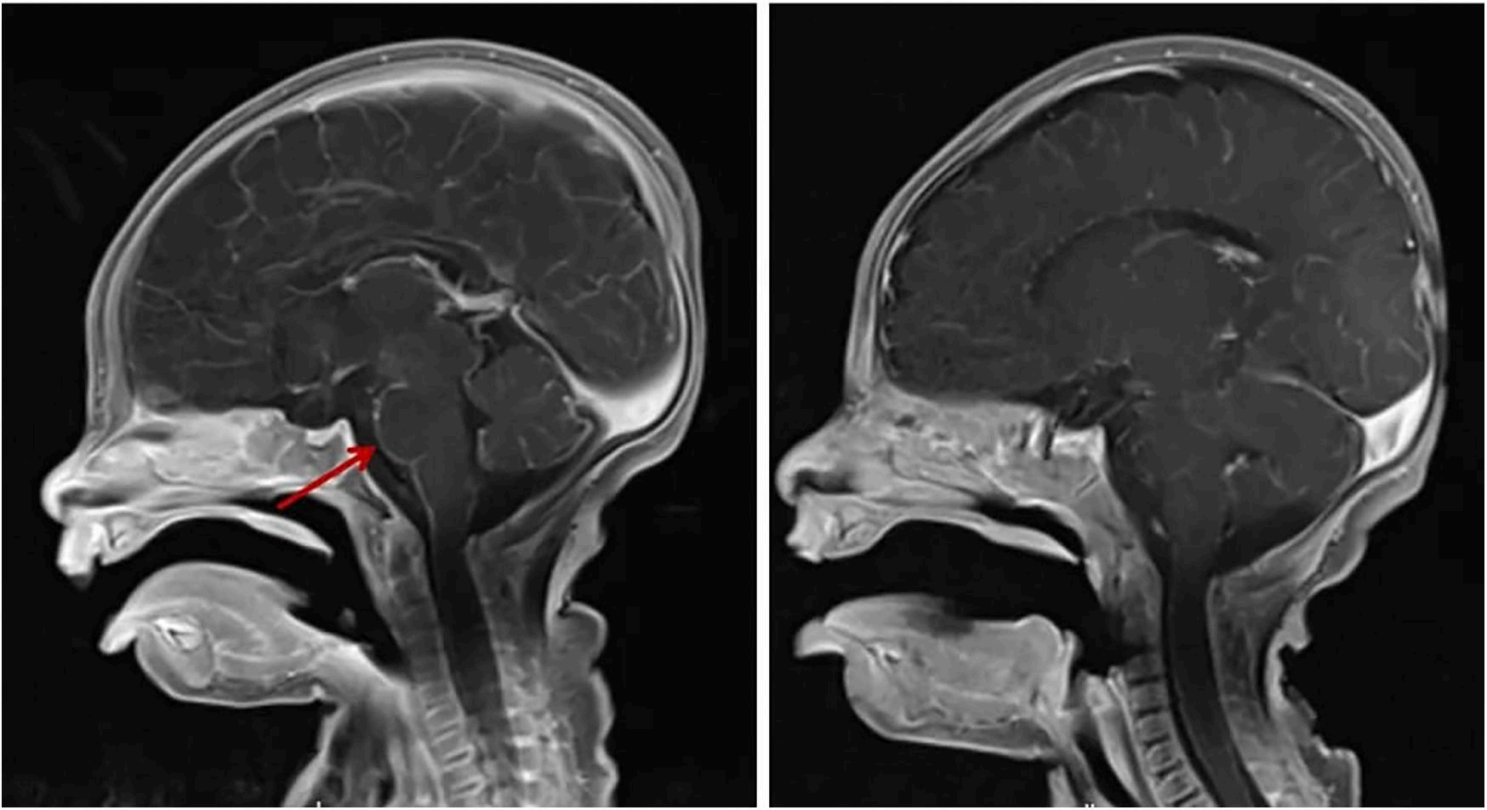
Sagittal views of post-contrast MRI show the difference between before (right) and after (left) treatment. The previously seen nodular and meningeal enhancements are improved.
